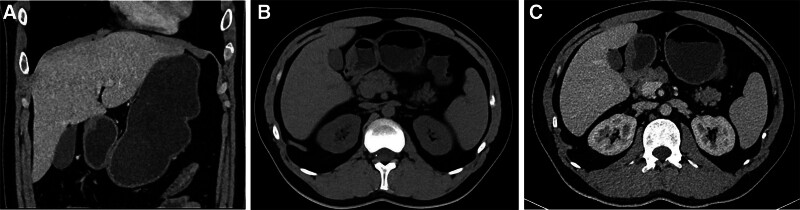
Abdominal enhanced computed tomography scan revealed an enhanced mass protruding into the stomach cavity.
